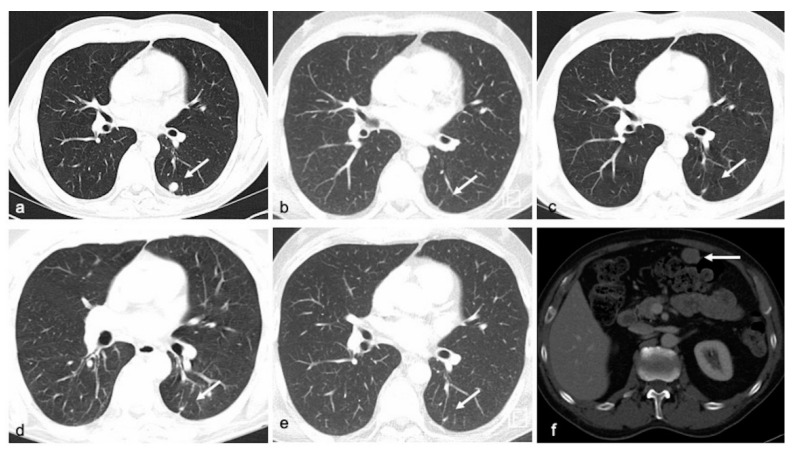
CT scans showing the patient’s (ID 6) response to treatment with dabrafenib/trametinib (a) Axial CT images of lung demonstrate a secondary lesion (target lesion) in left lower lobe in baseline examination. Patient also has known osseous metastases (nontarget lesions). (b–d) 3, 6, and 9 months after therapy, there is still no evidence of the pulmonary nodule, the osseous metastases remain unchanged, in summary corresponding to partial response. (e,f) 13 months after therapy, there is still no evidence of the pulmonary nodule, but a new mesenteric implant was found, suggestive of progressive disease, which later was confirmed to represent metastatic tissue.
